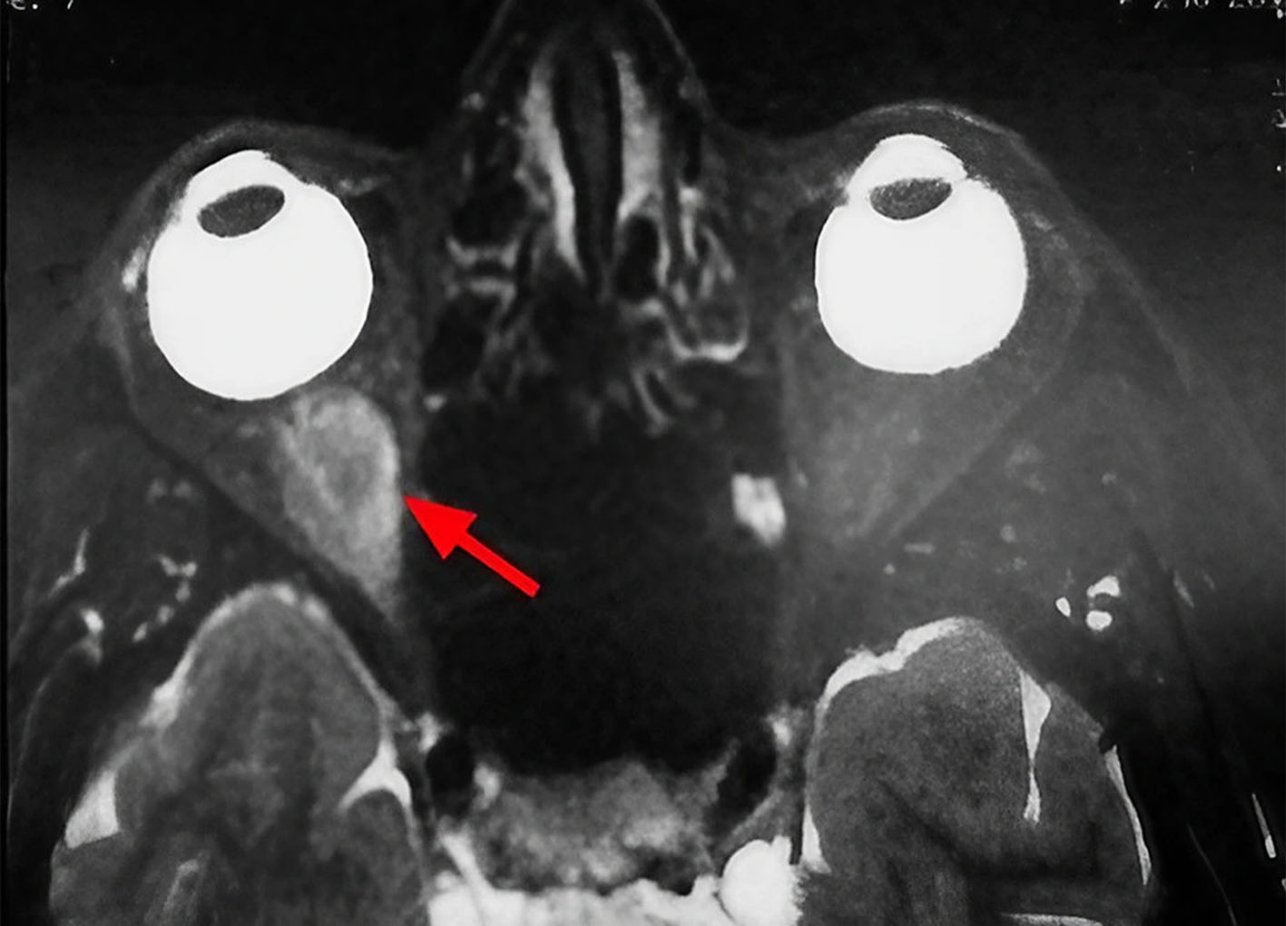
Sagittal section of orbital MRI showing a mass with high signal on a T2 weighted image (red arrow).
